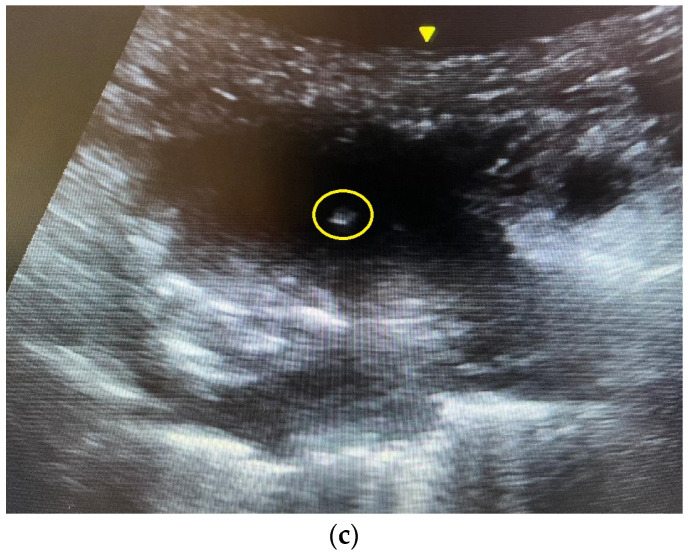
(a) A computed tomography image showing a right upper lobe Pancoast tumor. The tumor closely abutted the chest wall, allowing ultrasound-guided percutaneous biopsy. The pathology was squamous cell carcinoma. (b) An ultrasound image of the Pancoast tumor. The tumor had soft tissue density with heterogeneous echogenicity. (c) The yellow circle indicated the position of the needle tip inside the tumor. Several in and out motions were made with the needle, and the needle tip’s position was traced inside the tumor under real-time ultrasound guidance.
